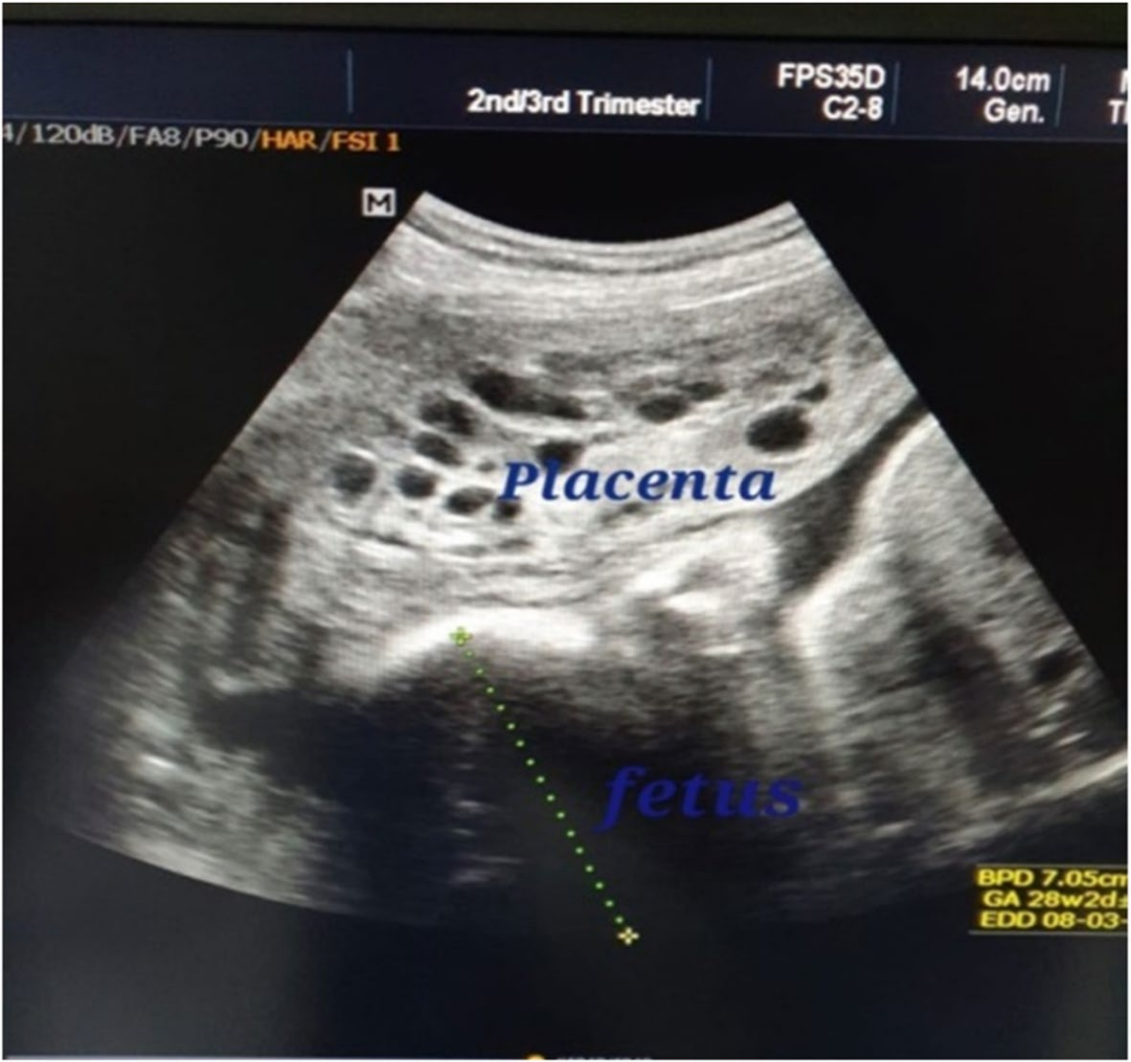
Ultrasound picture of pregnancy of the case (Z) at 28 weeks showing normal fetus with multiple variable-sized vesicles that cannot be separated from another placenta. The fetus is looking morphologically normal
